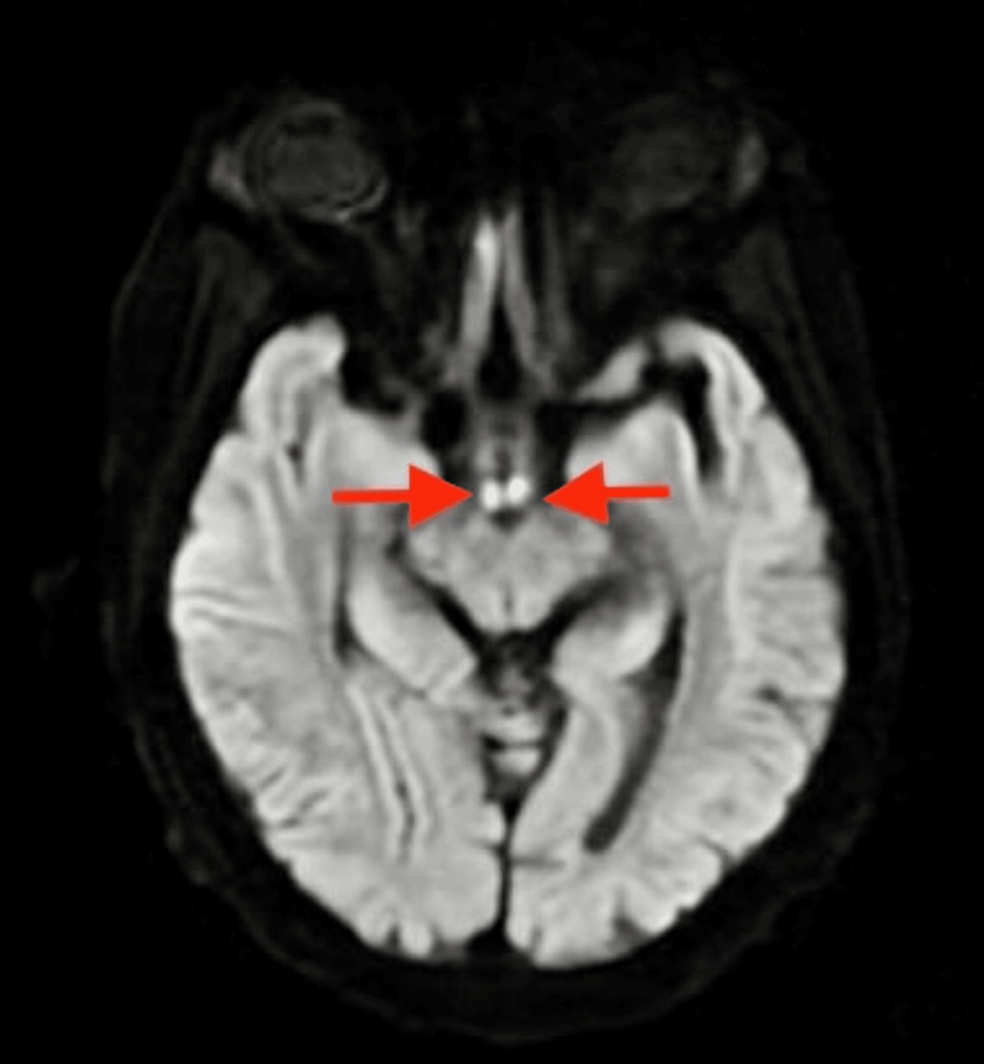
Non-contrasted MRI, axial view, DWI highlighting bright areas of high signal intensity affecting the mammillary bodies bilaterally (red arrows). DWI: Diffusion-weighted imaging
